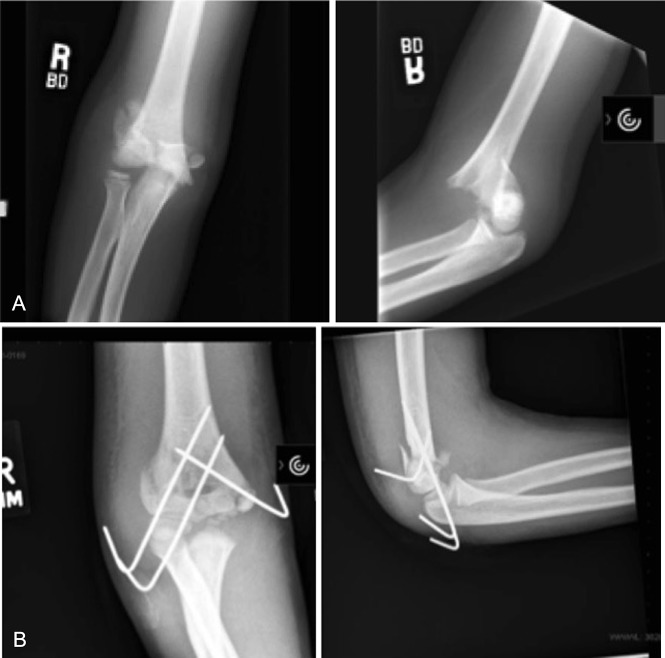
A, AP and lateral radiographs of a 9-year-old boys with a T-type distal humerus fracture. On initial glance, it resembles a regular extension-type supracondylar humerus fracture. B, AP and lateral radiographs after percutaneous fixation of the pediatric T-type distal humerus fracture.
